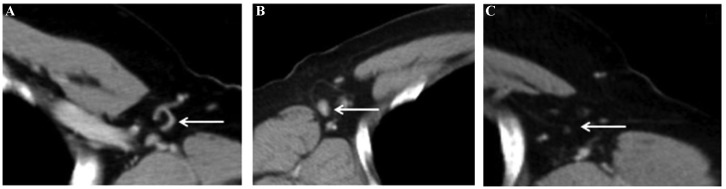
CT images showing (A) fat-, (B) clear-and (C) obscure-type axillary lymph nodes. CT, computed tomography.
